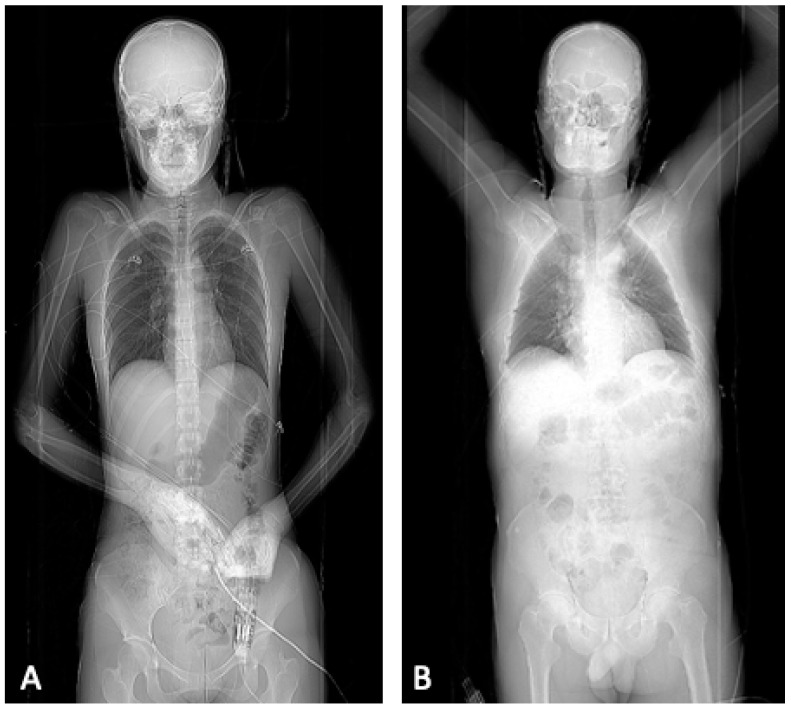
Example for the different positions of the patient during the scout varying between the evaluated computed tomography protocols. Image (A) shows the scout obtained for protocol A and B, whereas image (B) displays the scout of protocol C.
